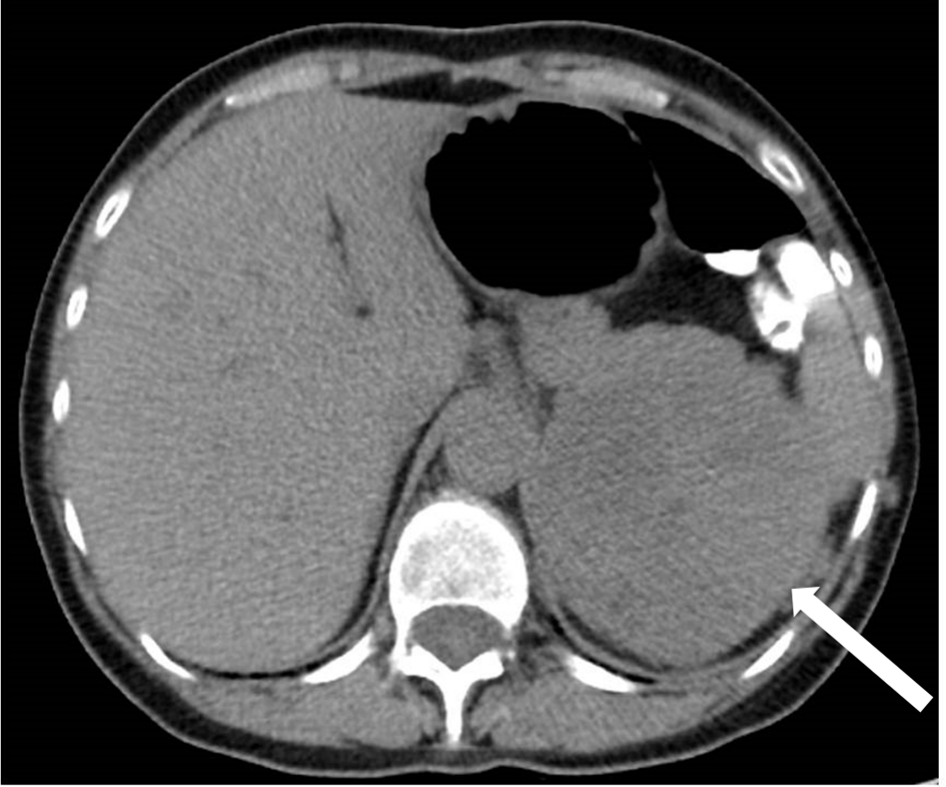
Left adrenal tumour, 9.5 cm in its largest diameter. Unenhanced computed tomographic scan. Native density 40 Hounsfield unit.
